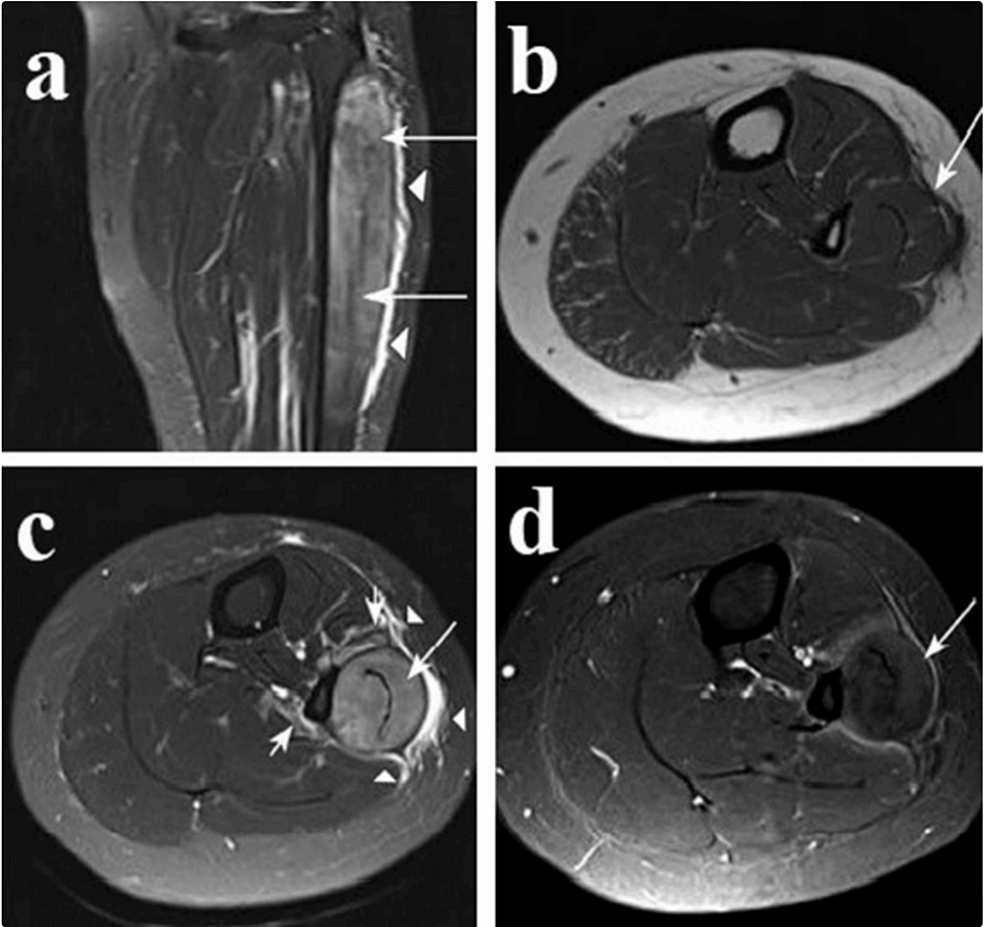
Isolated lateral compartment syndrome of a lower leg (a) Coronal fat-suppressed T2-weighted image shows a diffuse high signal intensity of the peroneus longus and brevis muscles (long arrows). Fluid signal intensity is noted within the surrounding deep and superficial fascial planes (arrowheads). (b) Axial T1-weighted image of the lower leg shows marked swelling of the peroneus longus and brevis muscles with peripheral convex indentation (arrow). (c) Axial fat-suppressed T2-weighted image shows a diffuse high signal intensity of the peroneus longus and brevis muscles (long arrows). Fluid signal intensity is noted within the surrounding deep and superficial fascial planes (arrowheads). Focal increased signal intensity suspected reactive change is noted in the extensor digitorum longus and soleus muscles around the peroneus longus muscle (short arrows). (d) Axial gadolinium-enhanced T1-weighted image shows heterogeneous enhancement within the affected muscles of the lateral compartment (arrow). Reprinted from Creative Commons under (CC BY-NC 3.0), copy and redistributable material [23].
